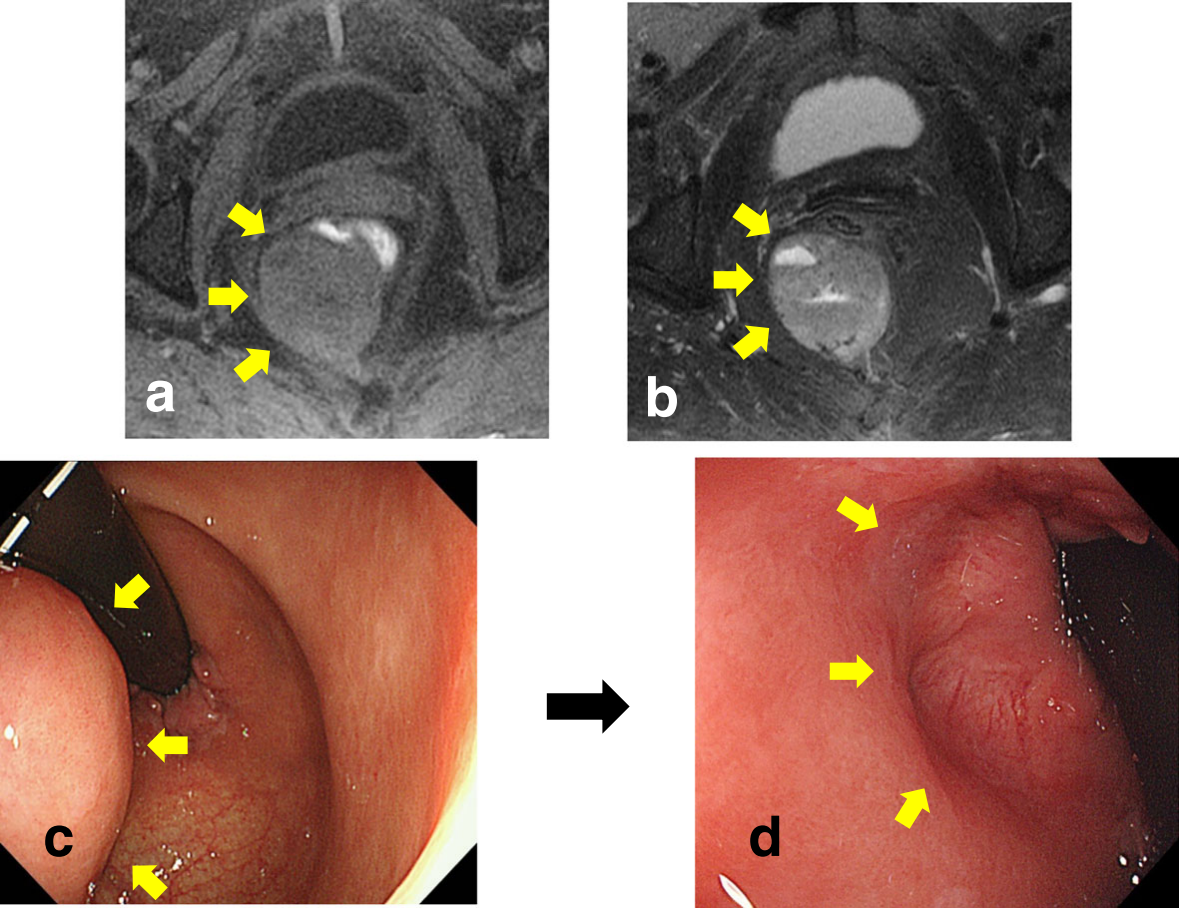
a T1-weighted MRI showed a low intensity tumor. b T2-weighted MRI showed a high intensity tumor. c Colonoscopic examination revealed a submucosal tumor at the posterior rectal wall, and that the distal tumor margin was 1 cm above the dentate line. d Colonoscopic examination revealed that the tumor markedly decreased after the treatment of imatinib
